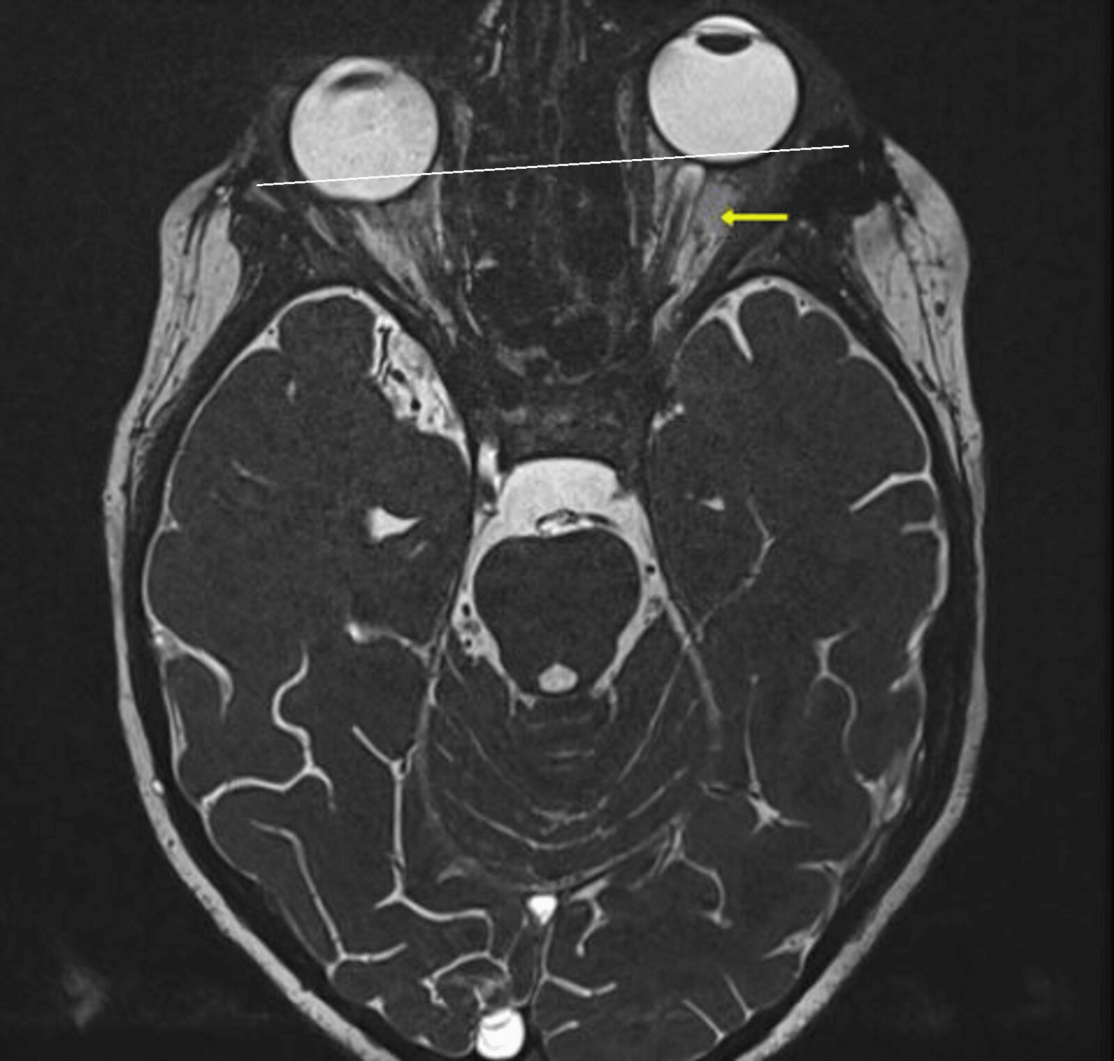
Axial T2 MRI section revealing a well-circumscribed intraorbital conal mass located at the level of the left superolateral orbital wall, with hypersignal on T2 (yellow arrow). It is responsible for grade I exophthalmos, as evidenced by the posterior third of the eyeball passing through the external bicanthal line (EBL). MRI, magnetic resonance imaging
